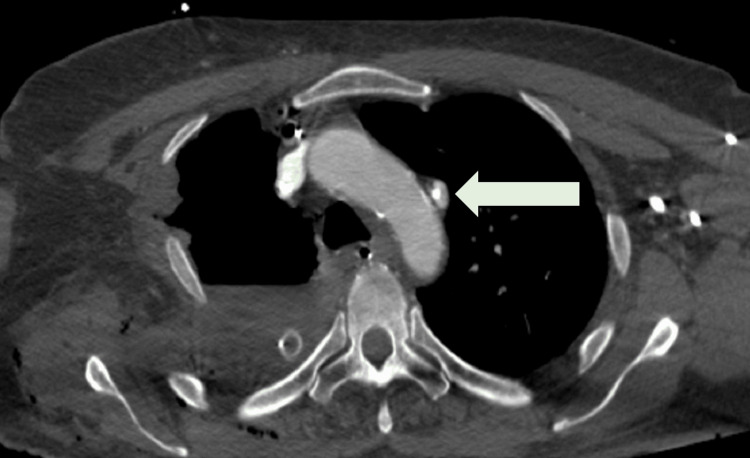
Axial (transverse) computed tomography angiogram slice demonstrating the anomalous venous return from the left upper pulmonary vein into the left internal jugular vein (arrow), with the central venous catheter seen within the aberrant vessel. This view confirms the unusual anatomy and explains the unexpectedly high oxygen saturation obtained from venous sampling.
